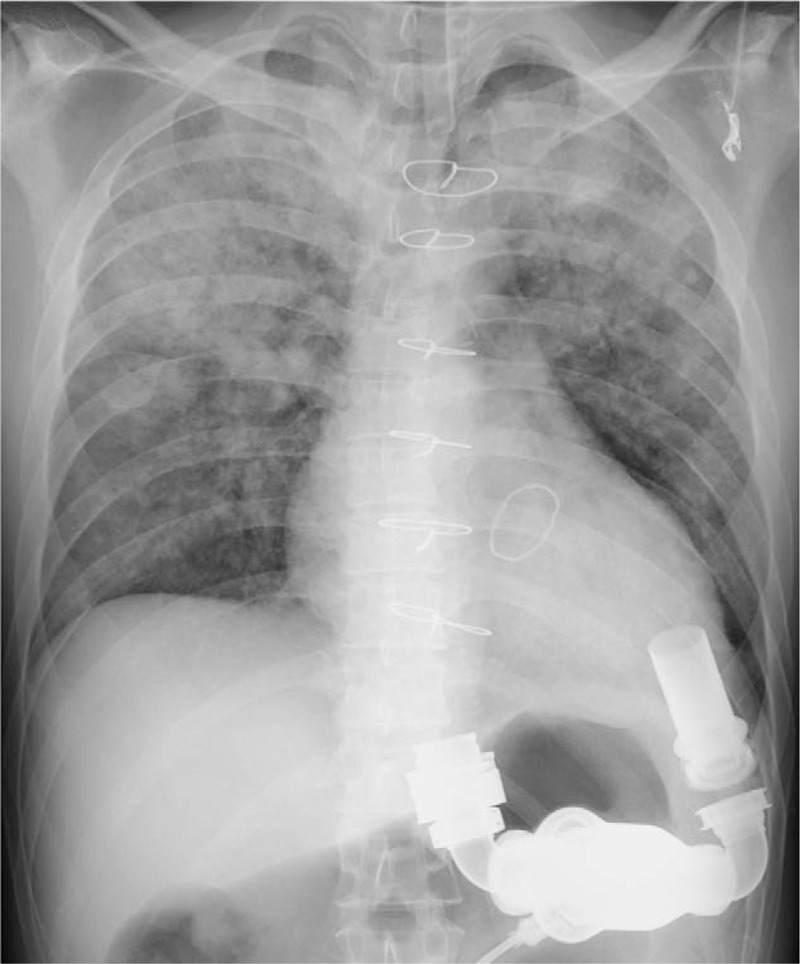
Chest X-ray showing diffuse bilateral infiltrates. There was no dislodgement of the LVAD.
